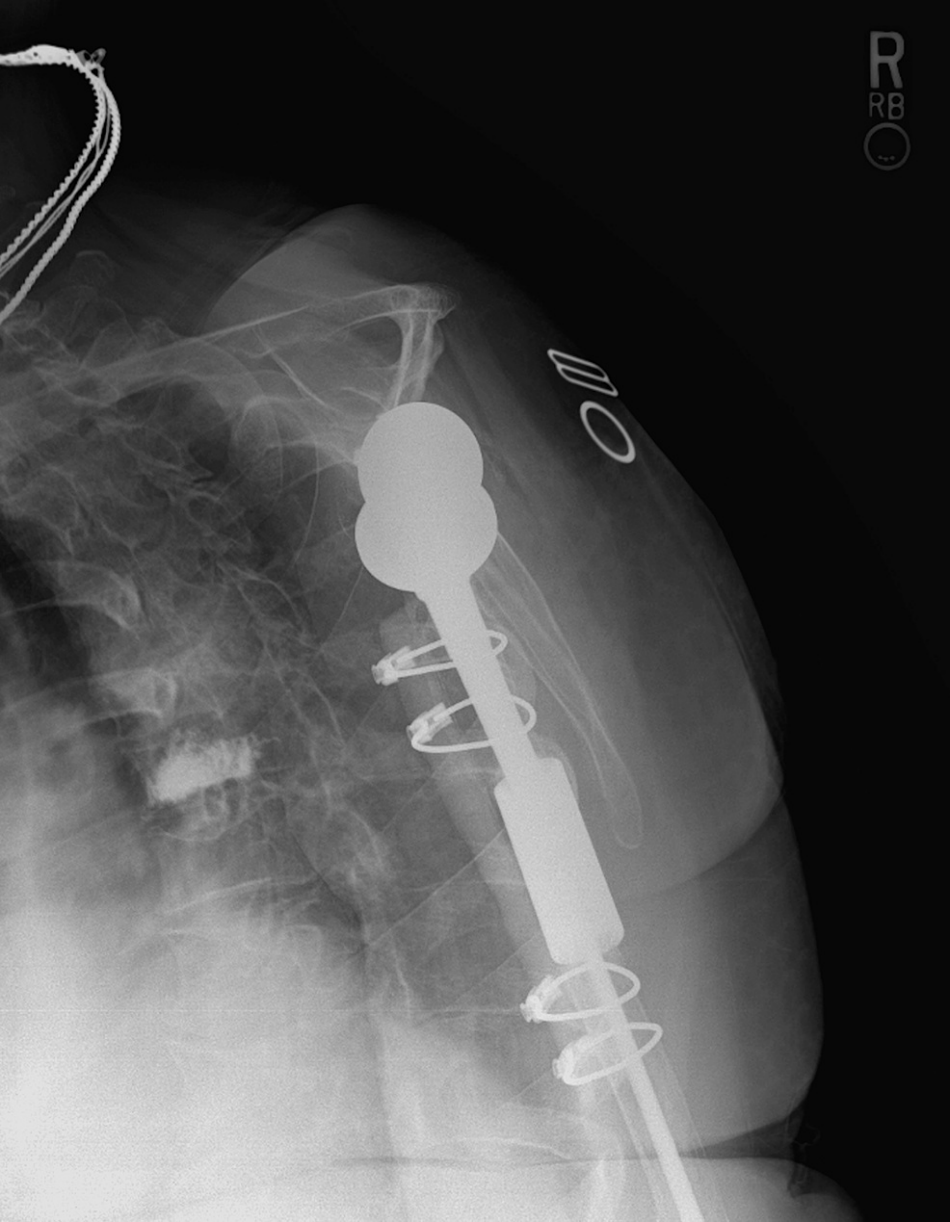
Lateral radiograph of the right shoulder at six-month follow-up.
